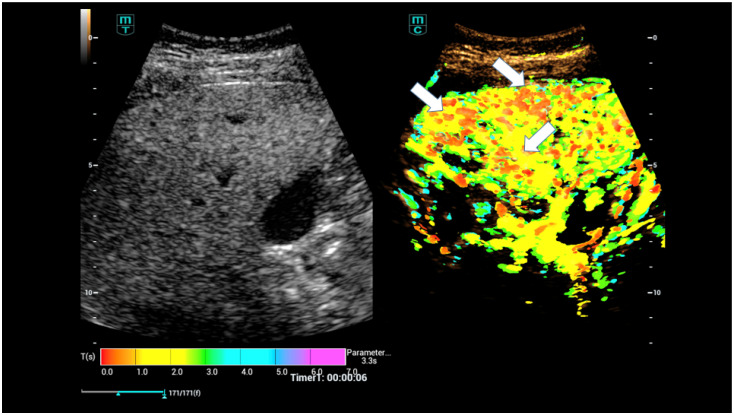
CEUS parametric evaluation after injection of 1.4 mL ultrasound contrast agent with irregular hyperenhancement of the liver and early nodular enhancement of high-flow haemangiomas (arrows) and micro-shunts with higher levels for time-to-peak (TTP) and area-under-the-curve (AUC).
